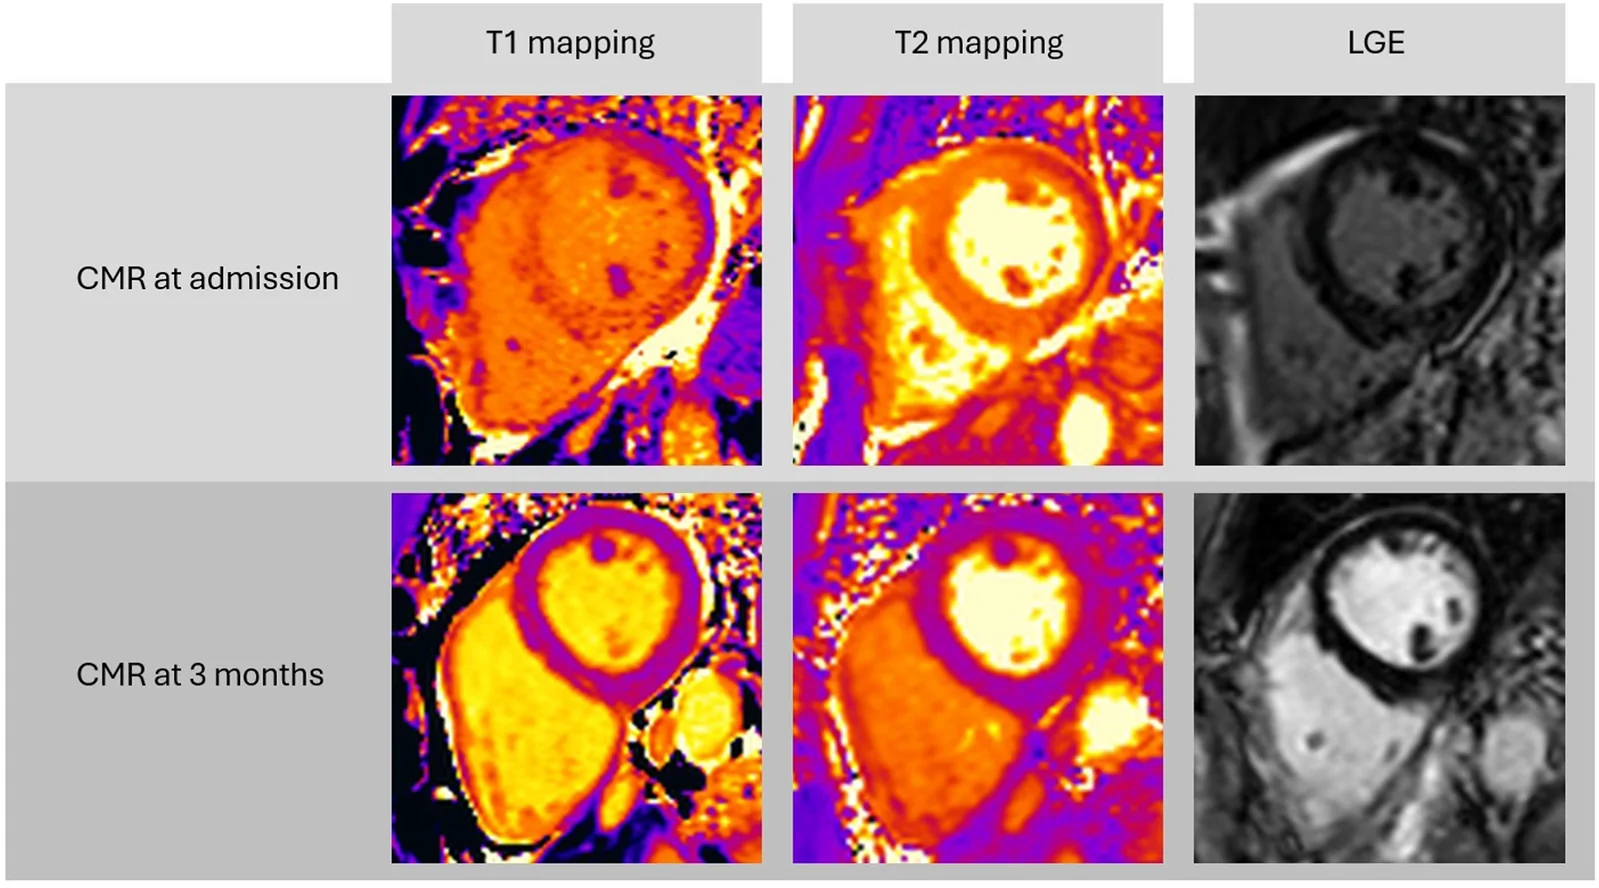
Cardiac magnetic resonance imaging at admission and at 3-month follow-up. T1 and T2 mapping. The initial scan shows abnormalities of the septum on both T1- and T2-weighted images. Late gadolinium enhancement is observed intramurally in the septal region and at the right ventricular insertion points. CMR = cardiac magnetic resonance; LGE = late gadolinium enhancement.
